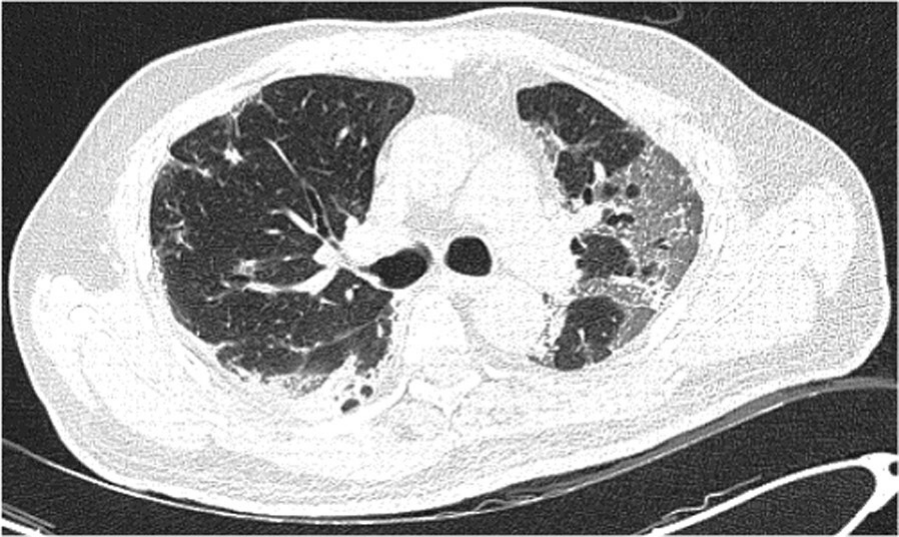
CT shows areas of consolidation and crazy paving in the upper lobes indicating early stage of lung involvement
